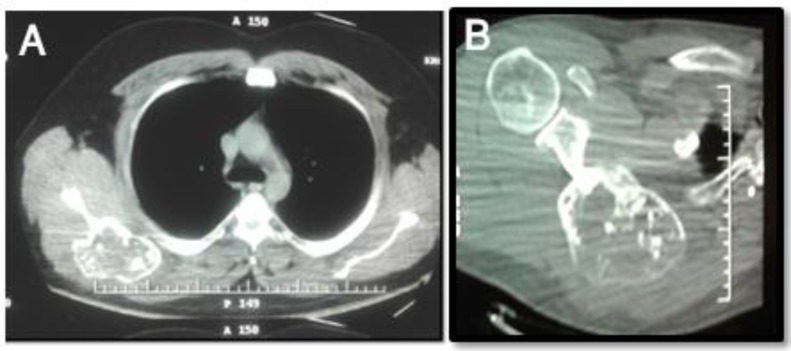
Computed Tomography images of right scapular recurred mass
